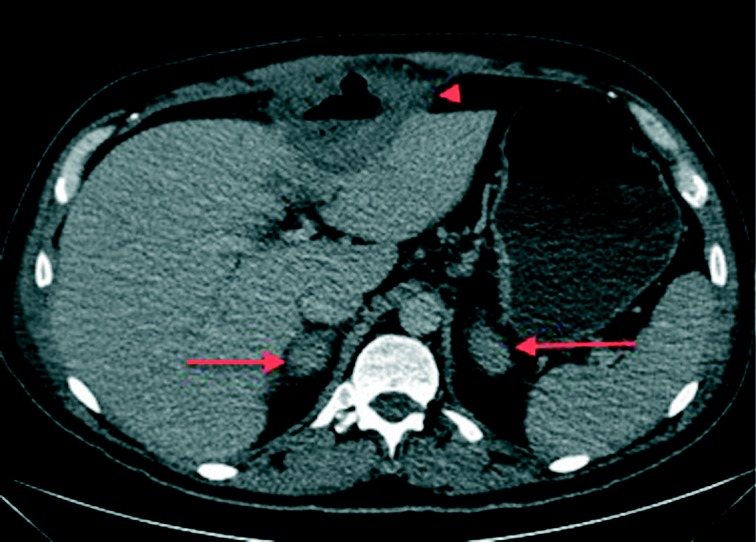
Axial view of CT adrenals revealing haemorrhagic adrenal glands (arrows) and drained abscess (arrow head).
